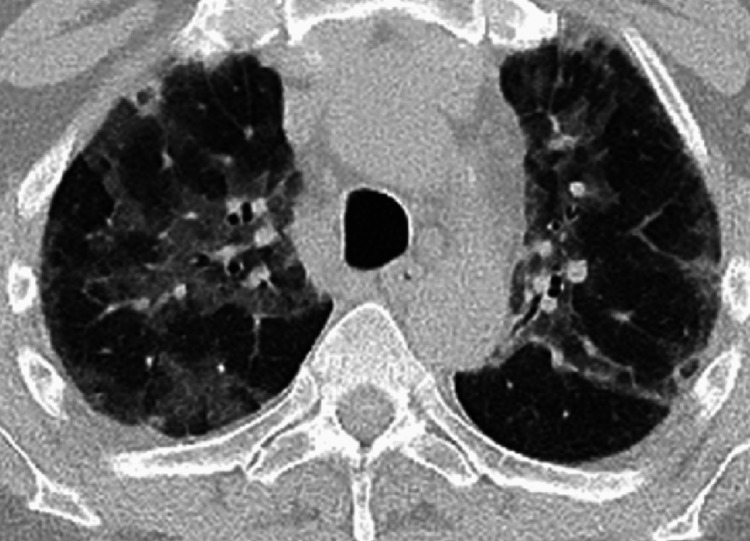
CT Chest without contrast
